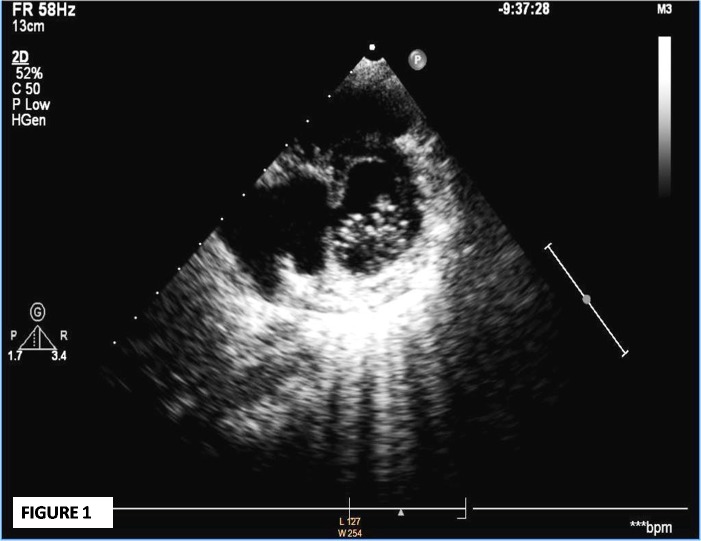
Figure 1:Parasternal short axis view at mitral valve level demonstrating a large aneurysmal sac on left of image with echo dropout at inferior septum.
